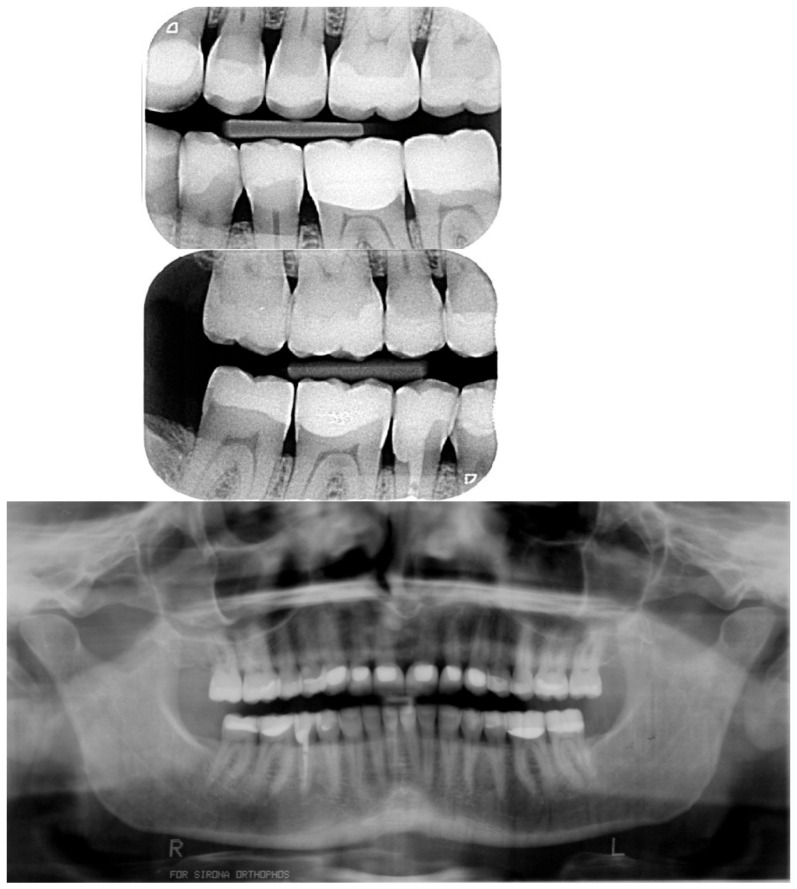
Radiographs after delivery of the restorations. All the teeth kept their vitality.
